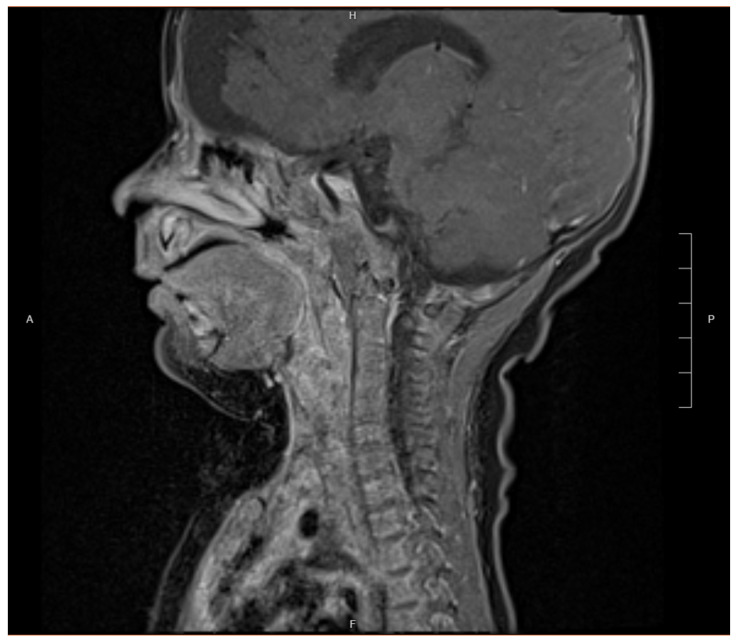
Dixon sagittal post-MRI image demonstrating enhancing mass along left prevertebral soft tissue in retropharyngeal space after resection and nine cycles of VAC chemotherapy.
